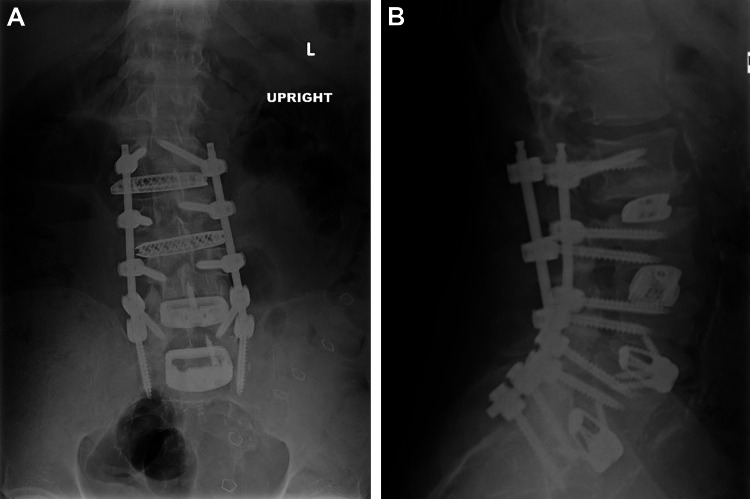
Postoperative (A) posterior and (B) lateral lumbar radiographs. Radiographs demonstrate the placement of extreme lateral interbody fusion cages in the L2-3 and L3-4 disk spaces, as well as anterior lumbar interbody fusion cages at L4-5 and L5-S1, with bilateral screws and rods placed percutaneously from L2 to S1. The image is used with permission from Barrow Neurological Institute, Phoenix, Arizona.
